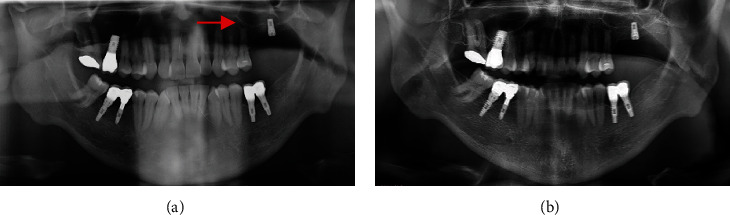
Case 1: (a) preoperative orthopantomography showing round, unilocular, radiolucent lesion developed in the region of lost dental implant (red arrow) and (b) postoperative orthopantomography.
